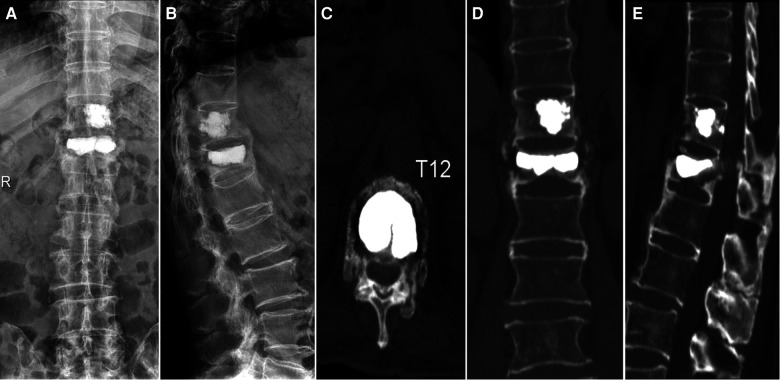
This patient received kyphoplasty. Three days later, anteroposterior (A) and lateral (B) x-ray showed the vertebral height and the Cobb's angle was restored. Traverse (C), coronal (D), and sagittal (E) CT showed intravertebral filling of the cavity without cement leakage.
